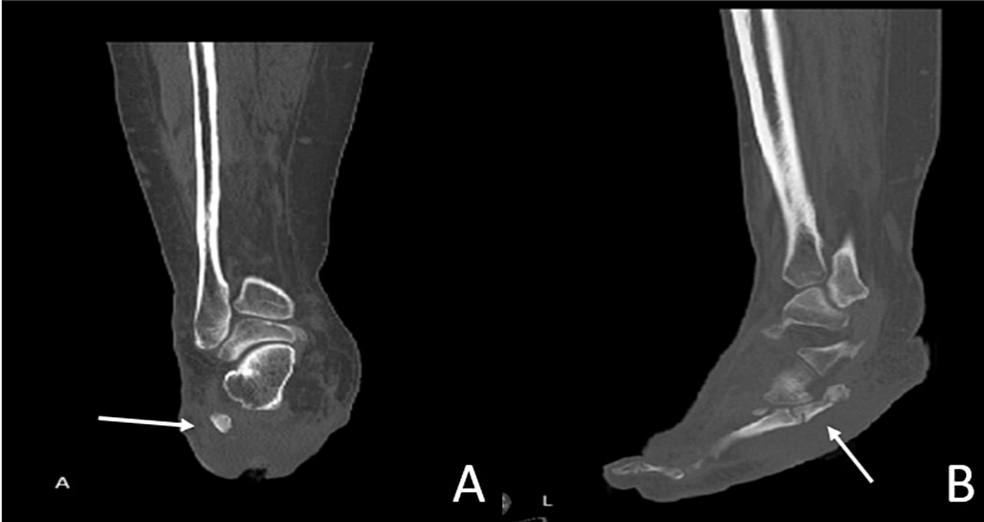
CT scan of the lower foot. (A) Coronal view of the right tarsal bones with an accessory bone. (B) Sagittal view of the right foot with an accessory bone connected to the base of the fifth metatarsal bone.
